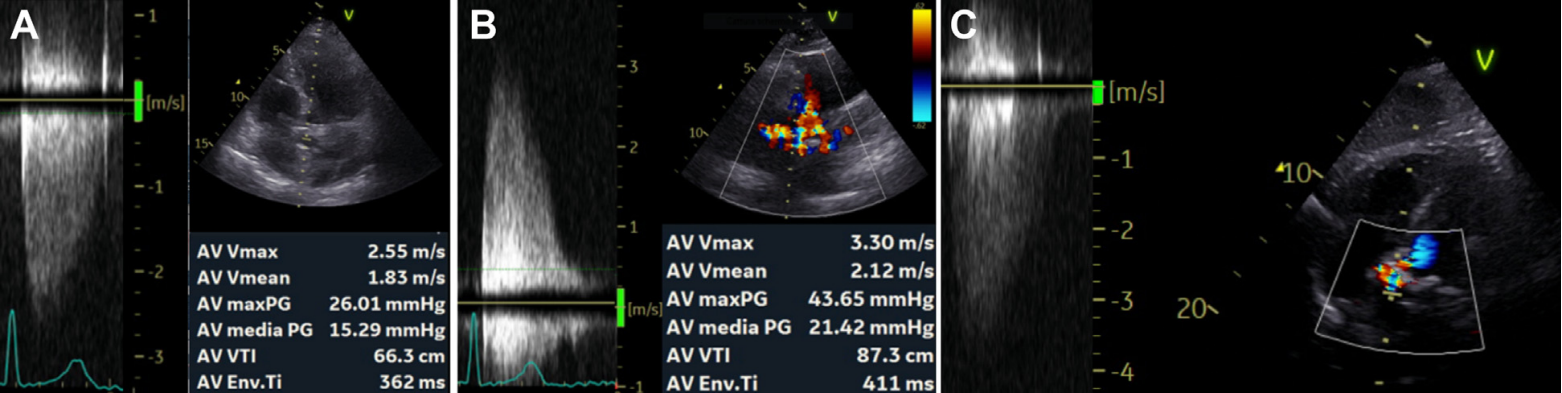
Moderate Aortic Valve Stenosis The right parasternal approach (B) and sometimes the subcostal view (C) can help to avoid the misclassification of aortic stenosis that is otherwise graded as mild from the apical view (A). AV Env.Ti = aortic envelope-time; AV maxPG = aortic peak gradient; AV media PG = aortic mean gradient; AV Vmax = aortic maximum velocity; AV Vmean = aortic mean velocity; AV VTI = aortic velocity-time integral.
